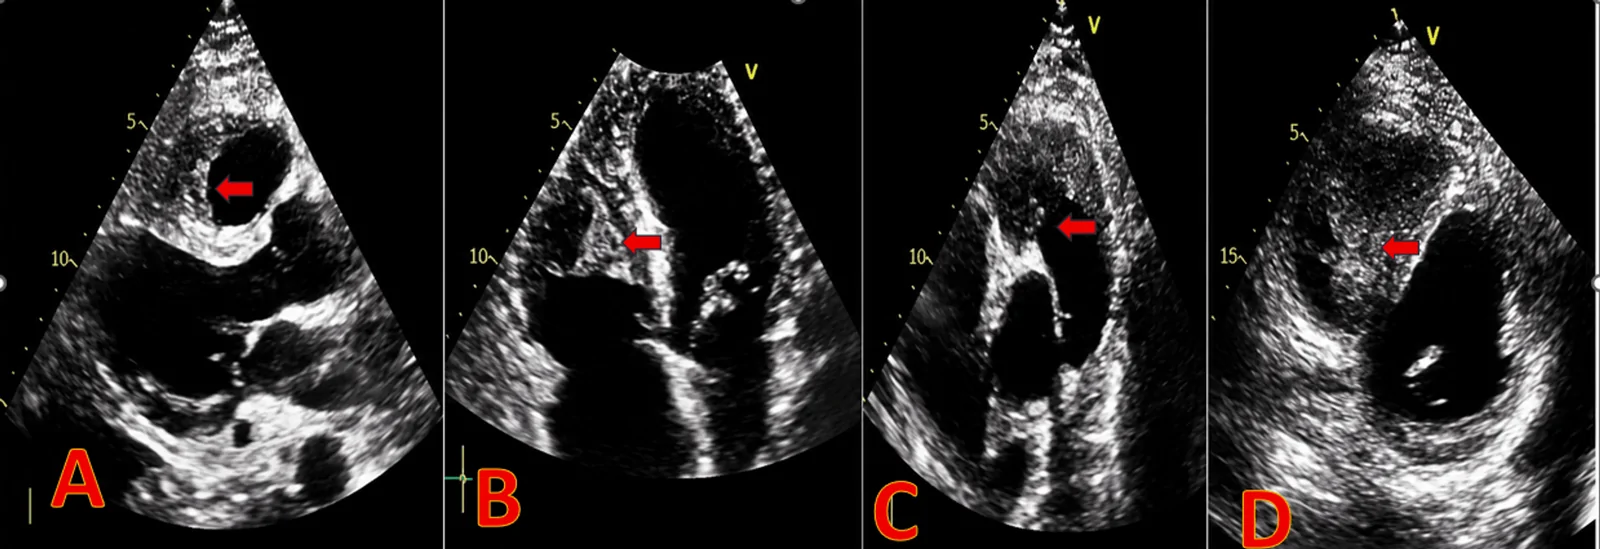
Transthoracic echocardiographic images. (A-D) Multiple views demonstrating an echogenic intracavitary right ventricular mass (red arrows), extending to the right ventricular outflow tract (pulmonary infundibulum) and attached to the septal leaflet of the tricuspid valve.
